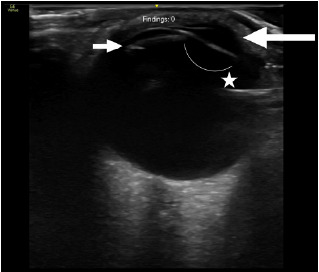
Point-of-care ultrasound demonstrating artificial lens dislocation. The large arrow denotes the anterior chamber of the eye, while the small arrow denotes the displaced lens. The correct anatomical position of the lens is denoted by the addition of the curved line adjacent to the star.
